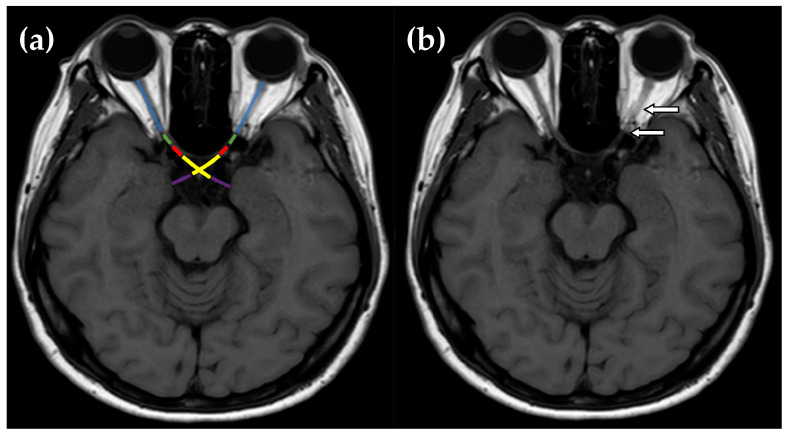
(a) Each orbital MRI scan was composed of five segments: orbital optic nerve (blue), intracanalicular optic nerve (green), intracranial optic nerve (red), optic chiasm (yellow), and optic tract (purple). (b) MRI T1W scan of a female patient diagnosed with double seronegative optic neuritis showed an orbital and intracanalicular optic nerve enhancement (arrows).
